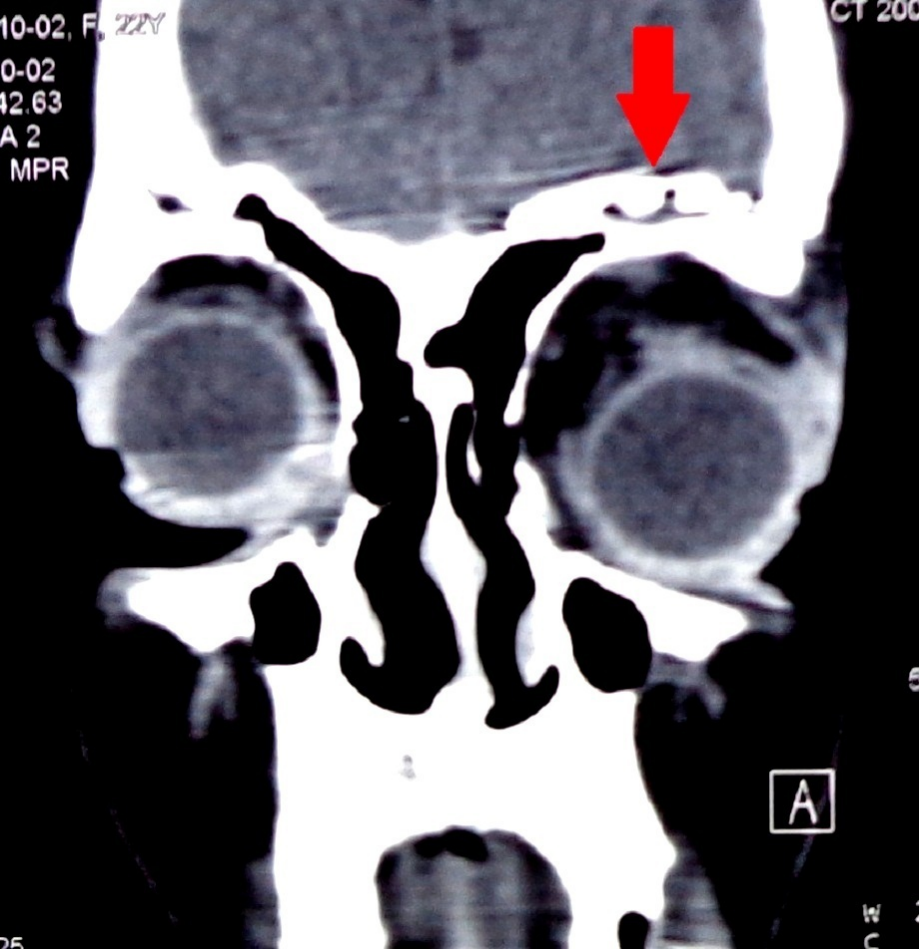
Post-operative CT scan coronal view (bone window) with metallic prosthetic covering the bony defect. CT: Computed tomography.
